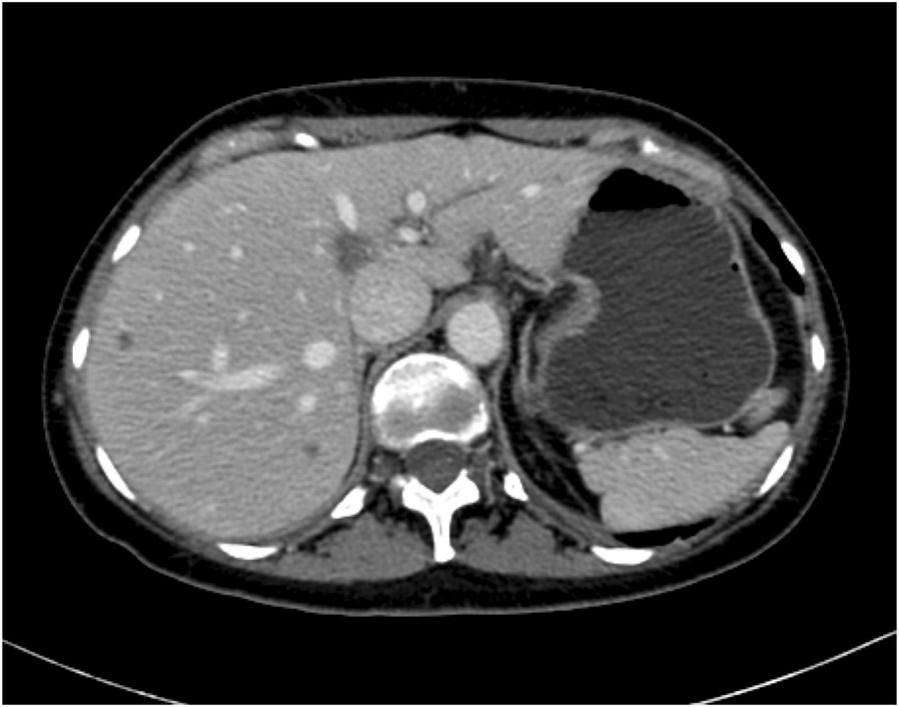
Hepatic lesions. In July 2011, CT scan showed occurrence of hepatic lesions
